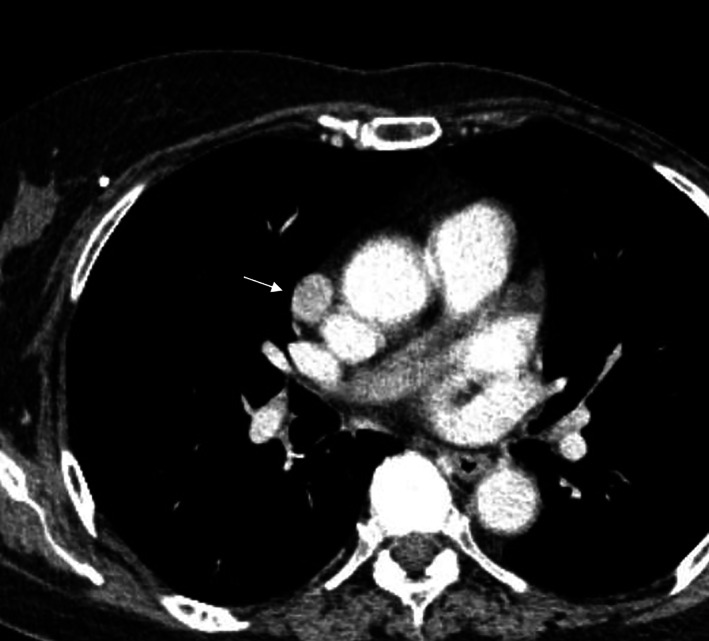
Contrast‐enhanced chest computed tomography revealed a well‐circumscribed tumor in the right lobe of the thymus (white arrow).
